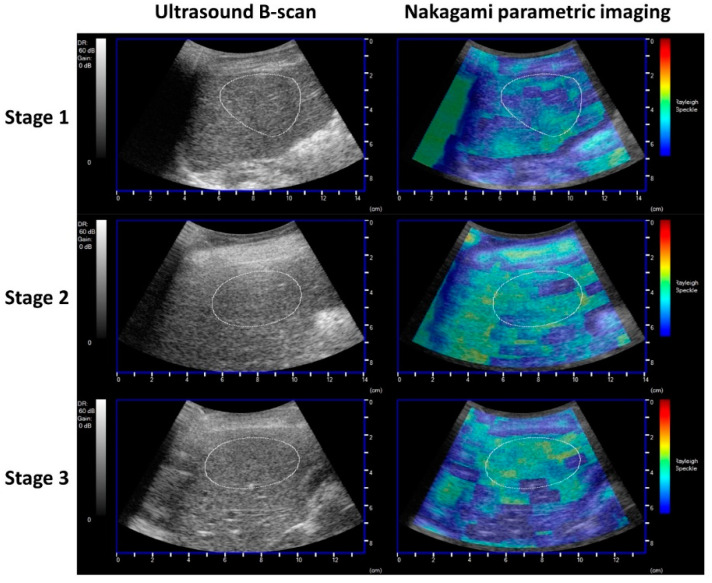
Ultrasound Grayscale B-mode and Nakagami parametric images of the liver in DMD patients at different ambulatory stages (stage 1: ambulatory stage, stage 2: early non-ambulatory stage, stage 3: late non-ambulatory stage). The brightness of ultrasound Nakagami imaging gradually increased with declining ambulatory stage of DMD. The NPI as a measure of hepatic steatosis was obtained by manually outlining the region of interest (ROI) (dotted line) on the Nakagami image for averaging the pixel values.
